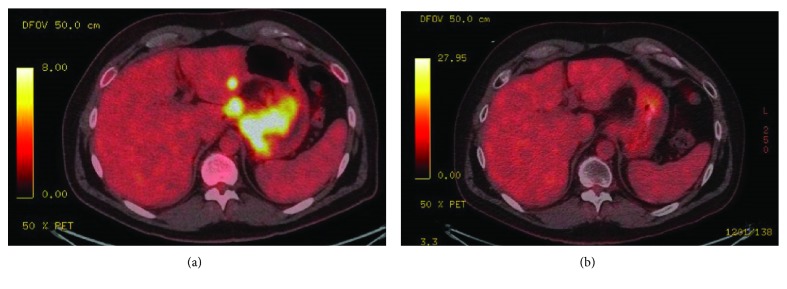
(a) Baseline axial fused PET/CT from May 2017 showing increased FDG uptake. (b) Axial fused PET/CT from March 2019 showing resolution of increased FDG uptake.
